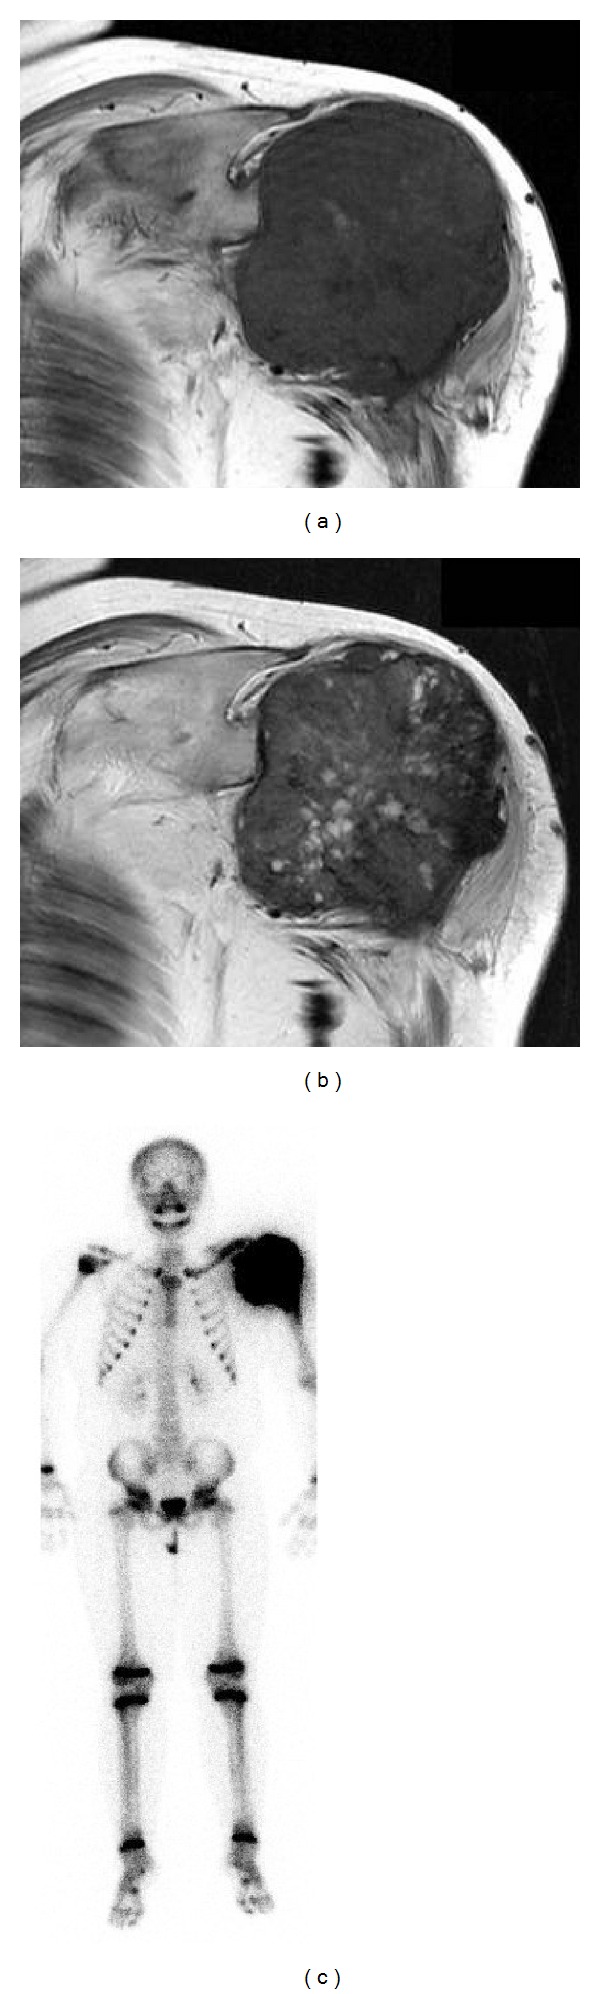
Coronal T1-weighted (a) and T2-weighted (b) MR images demonstrated a huge tumor in the proximal humerus. Whole body technetium-99m scan showed abnormal uptake in the left proximal humerus and surrounding area (c).
